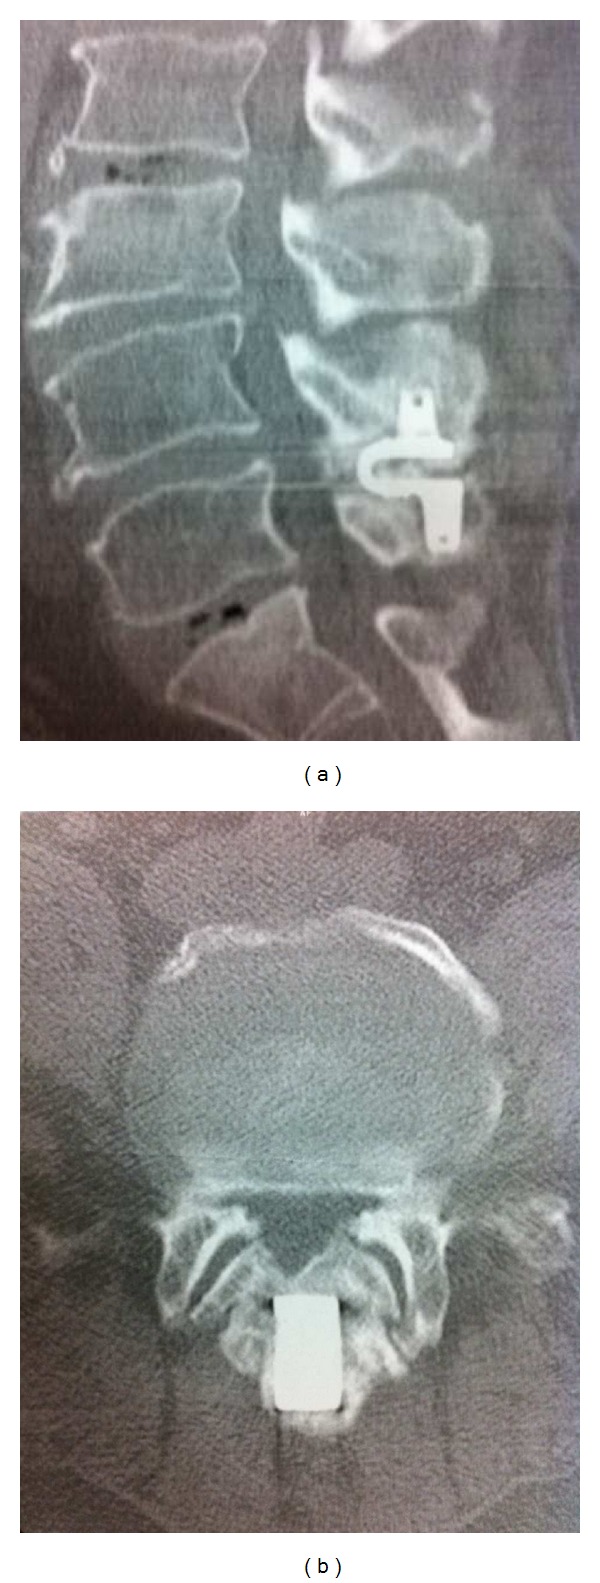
Preoperative CT bone scan showing heterotopic ossification of interspinous/interlaminar Coflex device.
